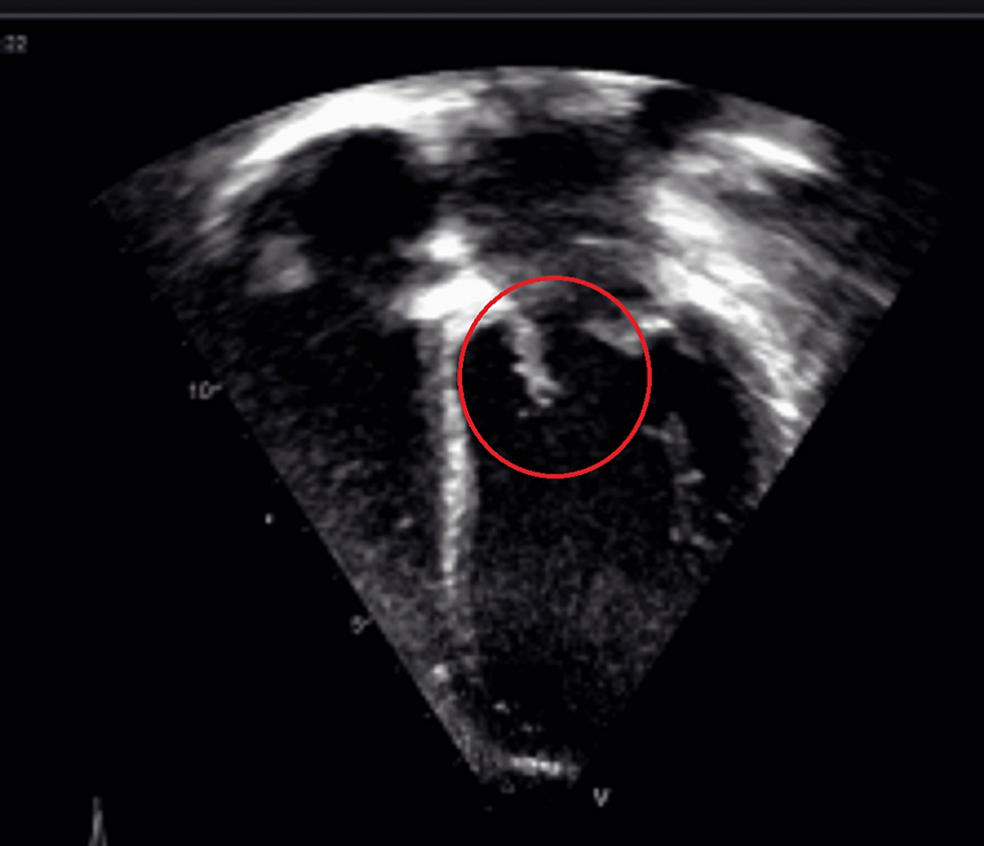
Echocardiography (Apical four-chamber view showing thick anterior mitral leaflet)
